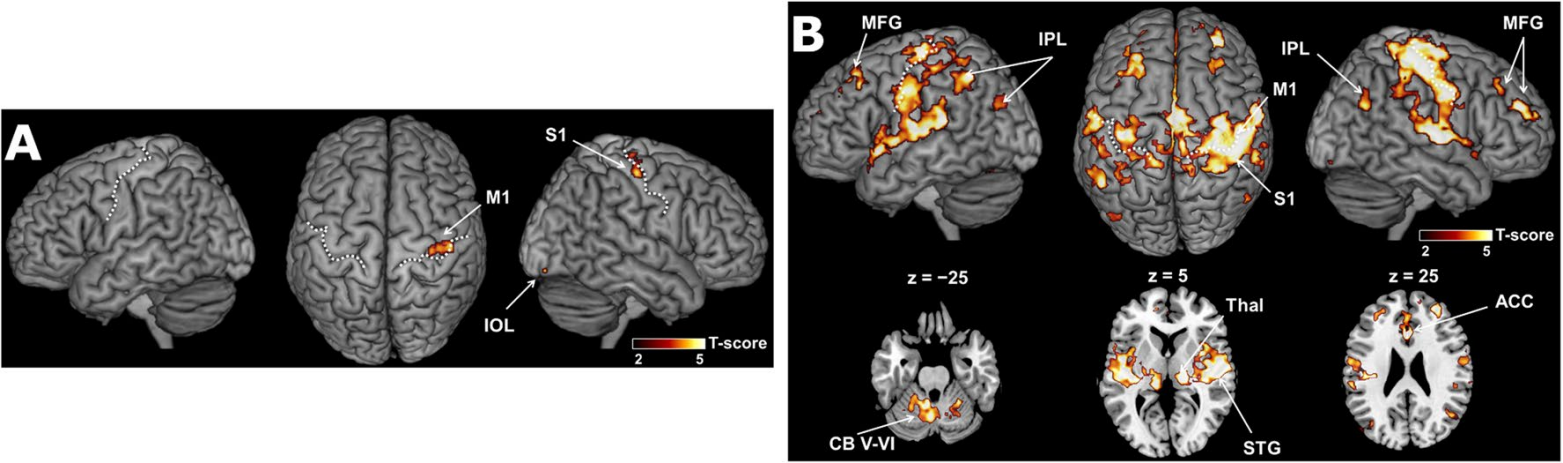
Learning related changes during execution and preparation. Linear increments in (A) execution-related and (B) preparation-related activity superimposed on the surface-rendered high-resolution magnetic resonance imaging of the template brain. The white dotted lines indicate the central sulcus. The level of statistical significance is set to *p* < 0.05, family-wise error corrected for multiple comparisons at the cluster-level. CB, cerebellum; IPL, inferior parietal lobule; MFG, middle frontal gyrus; S1, primary somatosensory cortex; M1, primary motor cortex; SMA, supplementary motor area; STG, superior temporal gyrus; Thal, thalamus; ACC, anterior cingulate cortex.
